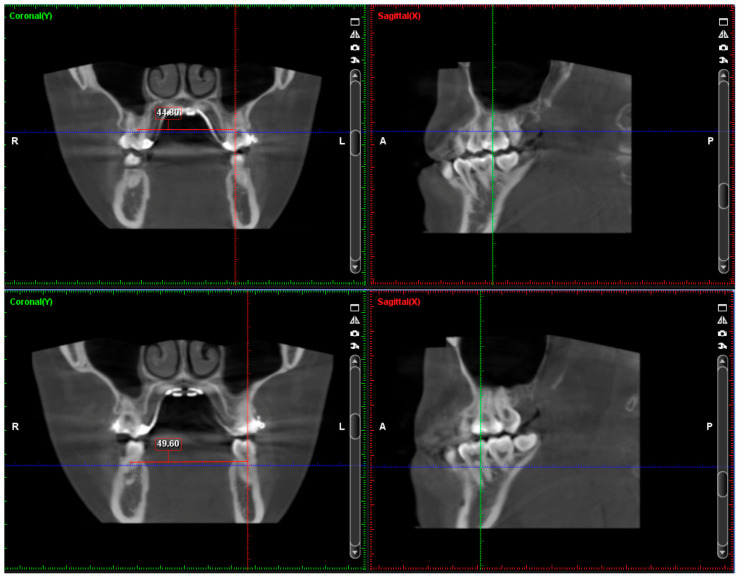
Pretreatment cone beam computed tomography (CBCT) images and measures. Inter-furcation distance of upper first molars was 44.8 mm, and inter-furcation distance of lower first molars was 49.6 mm. According to Yonsei Transverse Index (YTI), about 5 mm of maxillary expansion was needed. (Red line: Sagittal plane; green line: Coronal plane; blue line: Axial plane).
